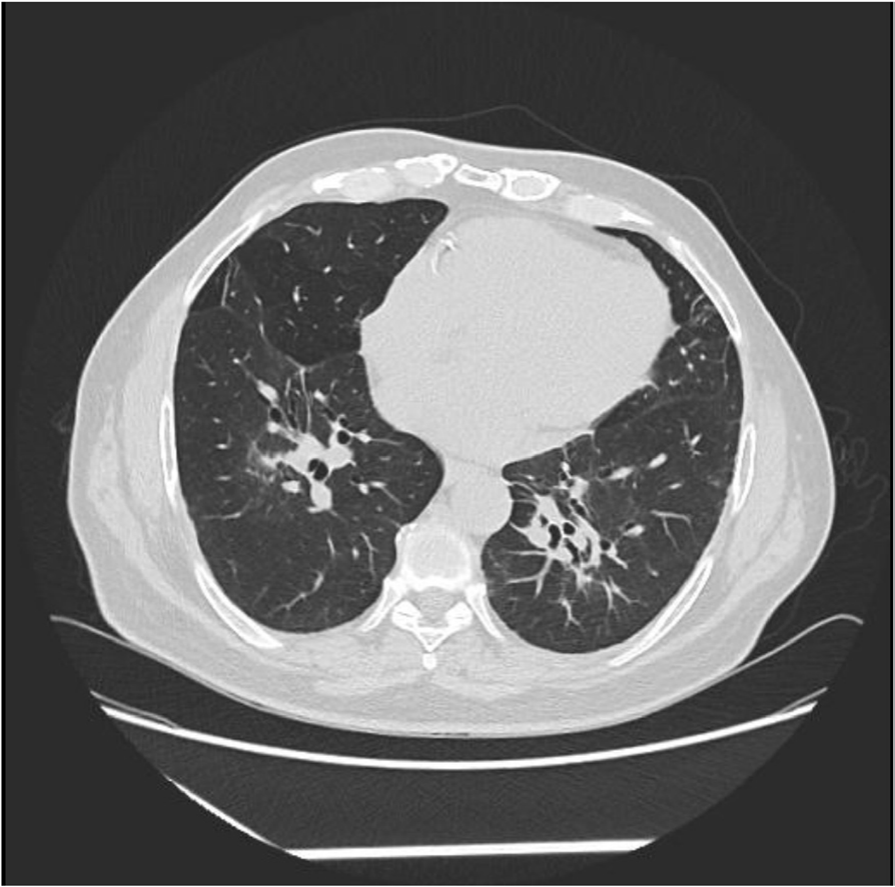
A repeated high-resolution computed tomography of the chest revealed the total subside of the previously noted diffuse pulmonary ground-glass opacities with patchy para-hilar consolidations
